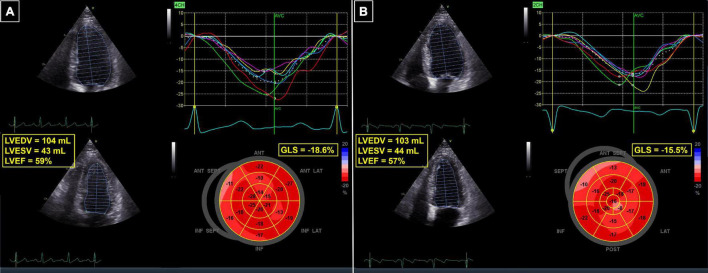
62-year-old patient with breast cancer undergoing chemotherapy with anthracyclines and radiotherapy. (A) Baseline echocardiogram, showing normal LVEF and GLS. (B) Follow-up echocardiogram 3 months after completion of therapy—the LVEF was still normal, but a 17% relative reduction in GLS revealed cardiotoxicity. GLS, global longitudinal strain; LVEDV, left ventricular end-diastolic volume; LVEF, left ventricular ejection fraction; LVESV, left ventricular end-systolic volume.
